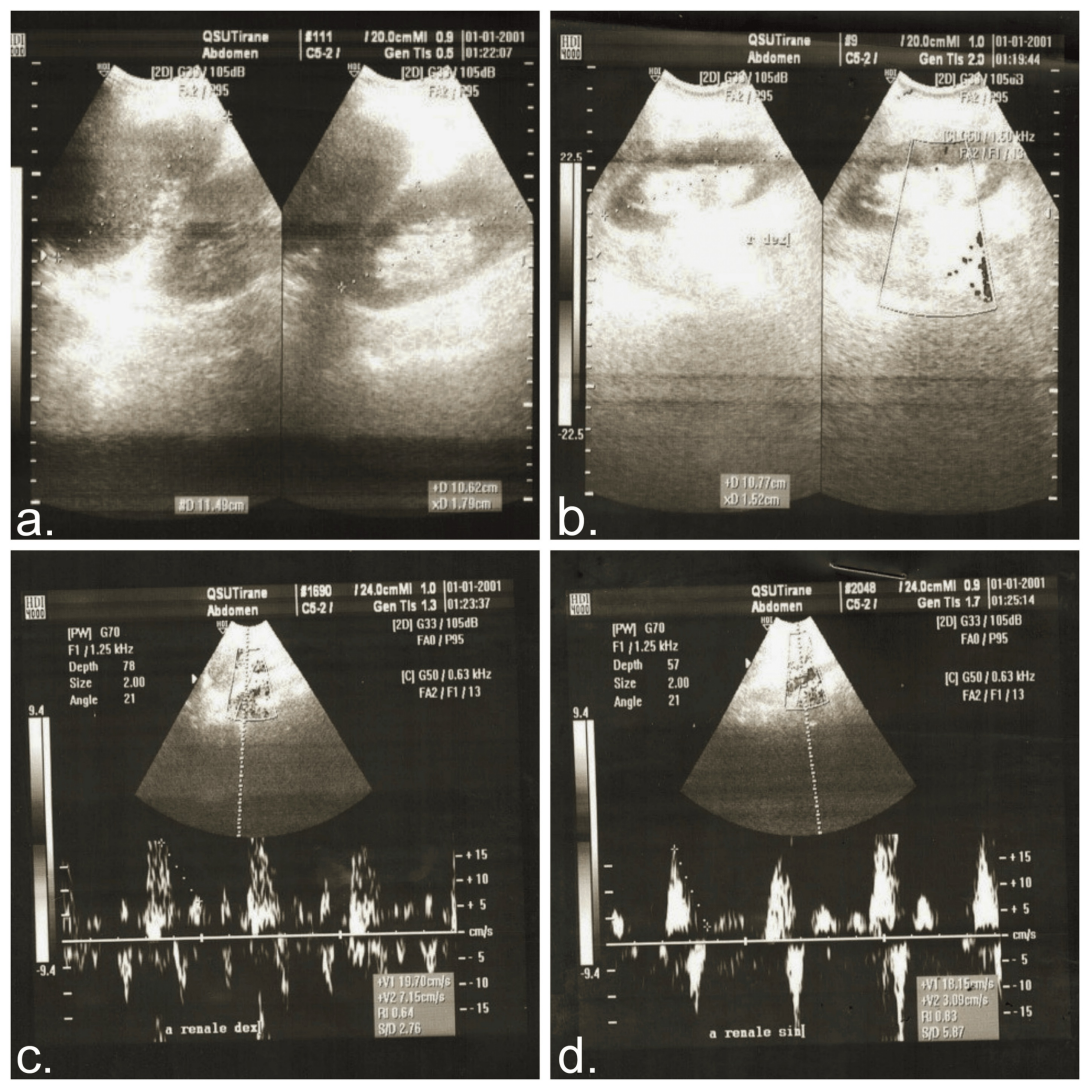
Renal ultrasound findings Left kidney (a) and right kidney (b) showing normal parenchyma. Right renal artery (c) and left renal artery (d) showing elevated peak systolic velocity, but normal renal blood flow
